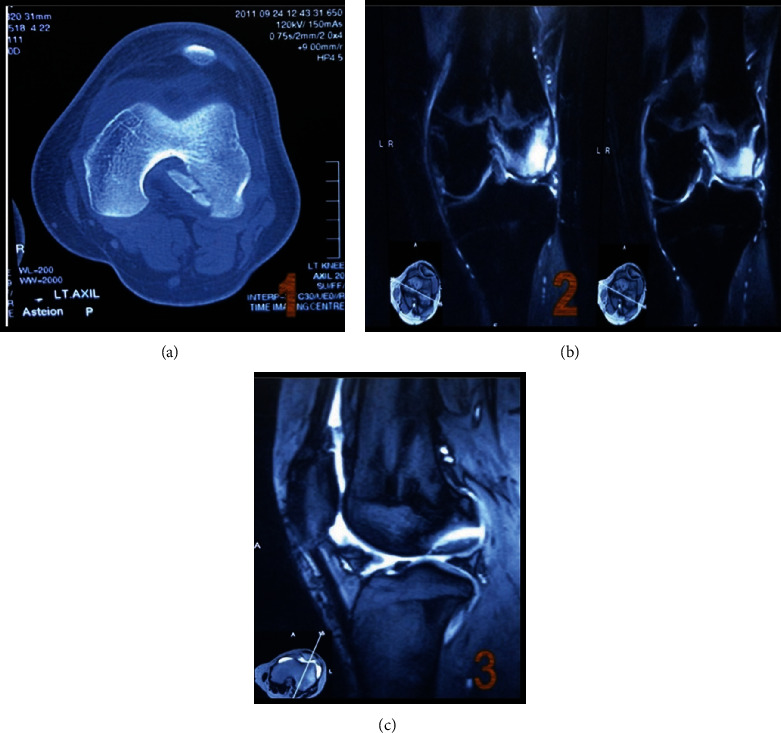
(a) CT scan notch view and (b, c) MRI image showing large defect at the posteromedial aspect of the lateral condyle femur.
